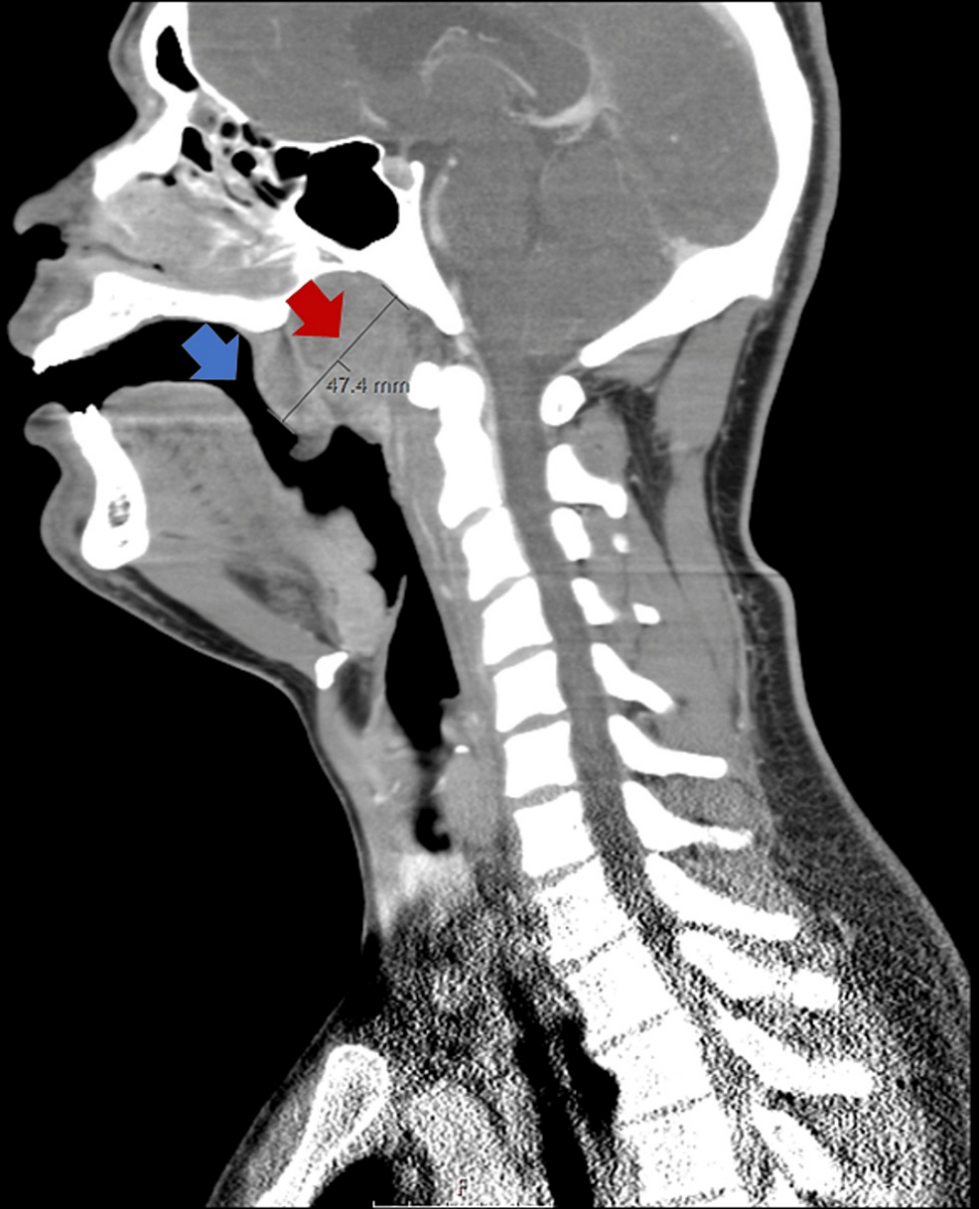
Neck CT scan sagittal view Diffuse swelling of Waldeyer’s tonsillar ring (red arrow). Nasopharyngeal airway narrowing (blue arrow).
